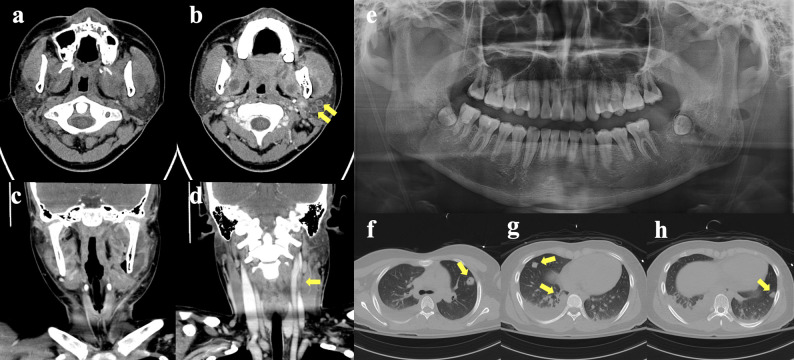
Initial assessment. a, b, c, d Contrast enhanced neck CT; multifocal abscess and myositis in both masticator spaces and masseter muscle. (arrows: acute thrombophlebitis in left EJV and facial vein) e Panoramic radiograph. f, g,h Chest CT; right pleural effusion. (arrows: septic emboli in both lung)
